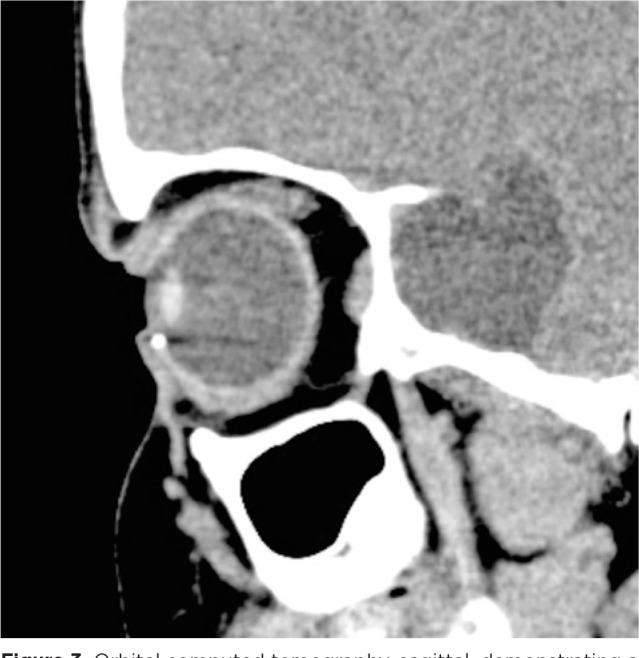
Orbital computed tomography, sagittal, demonstrating a metallic foreign
